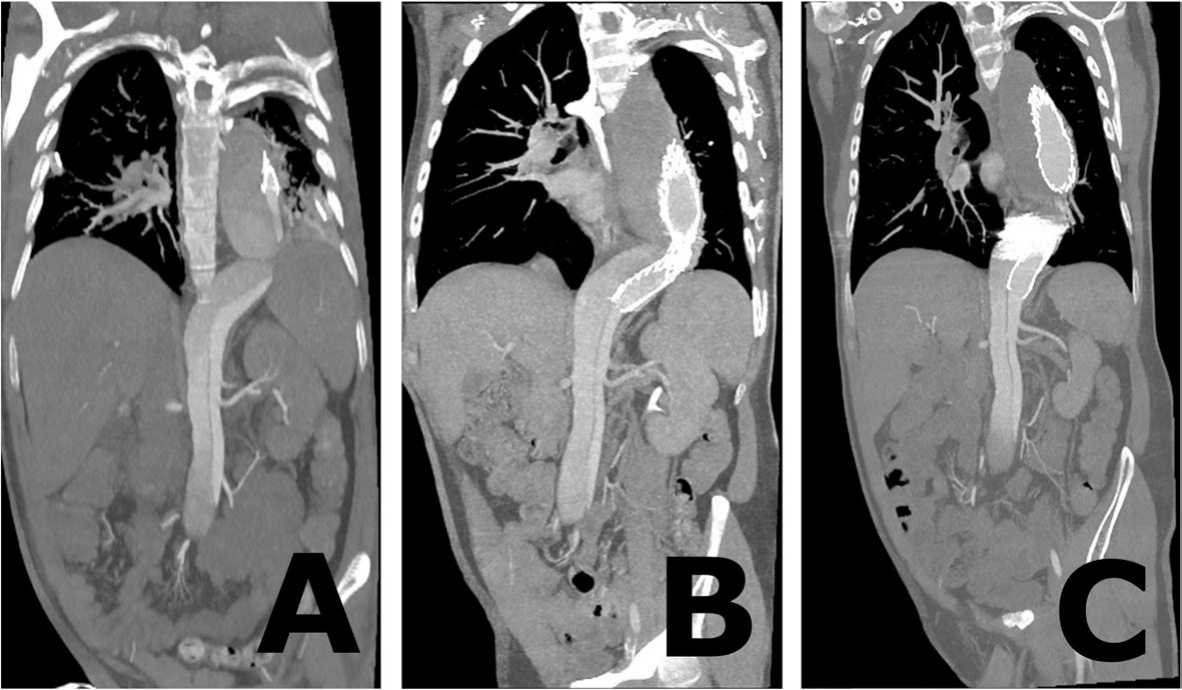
A Coronal CT image before extension TEVAR showing filling of the FL. B Coronal CT image after extension TEVAR showing continuous filling of the FL. C Coronal CT image after extension TEVAR and CP procedure showing significantly reduced filling of the FL
